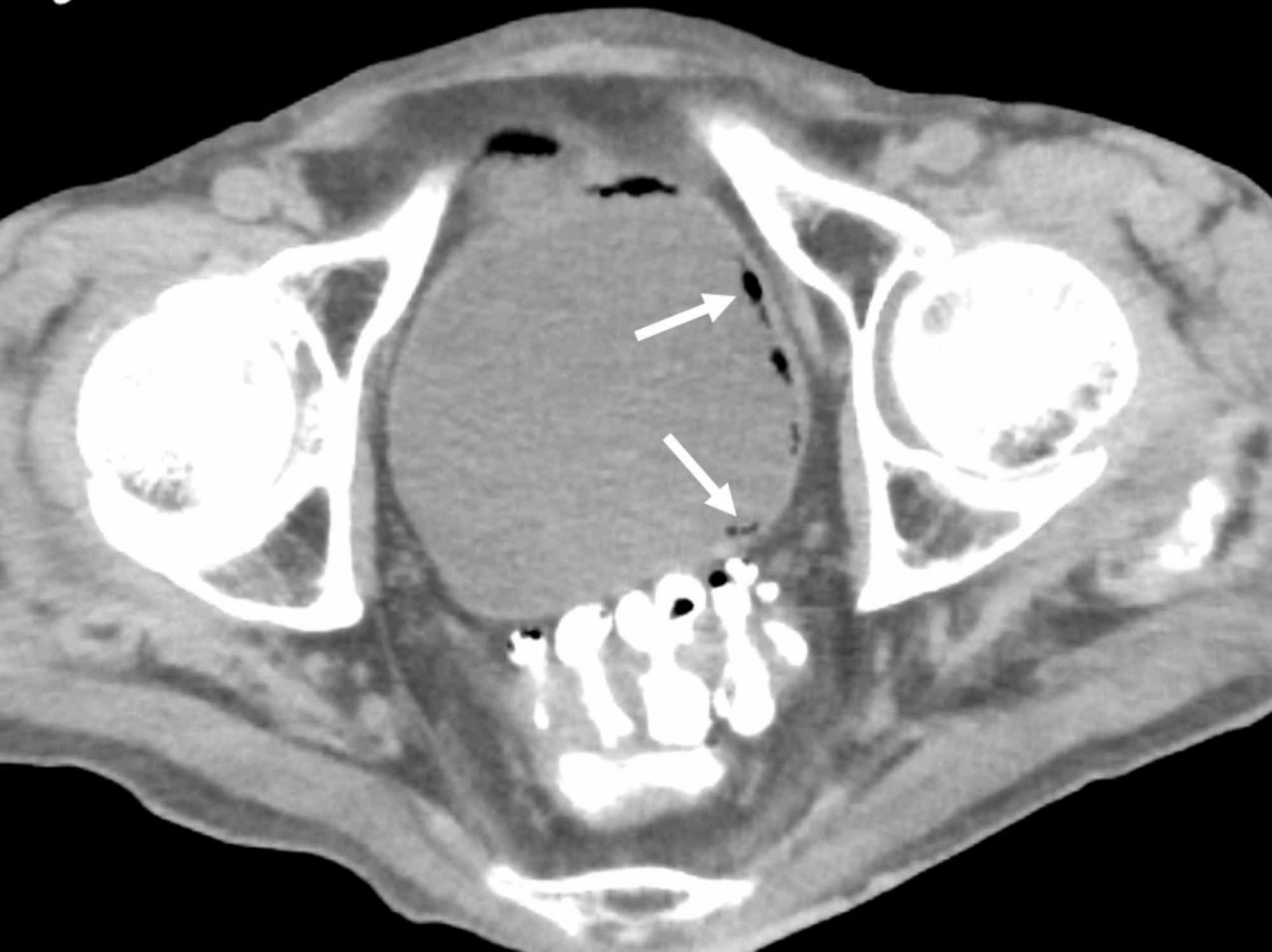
Computed tomography (CT) on the 81st hospital day CT revealed emphysematous cystitis (arrows).
